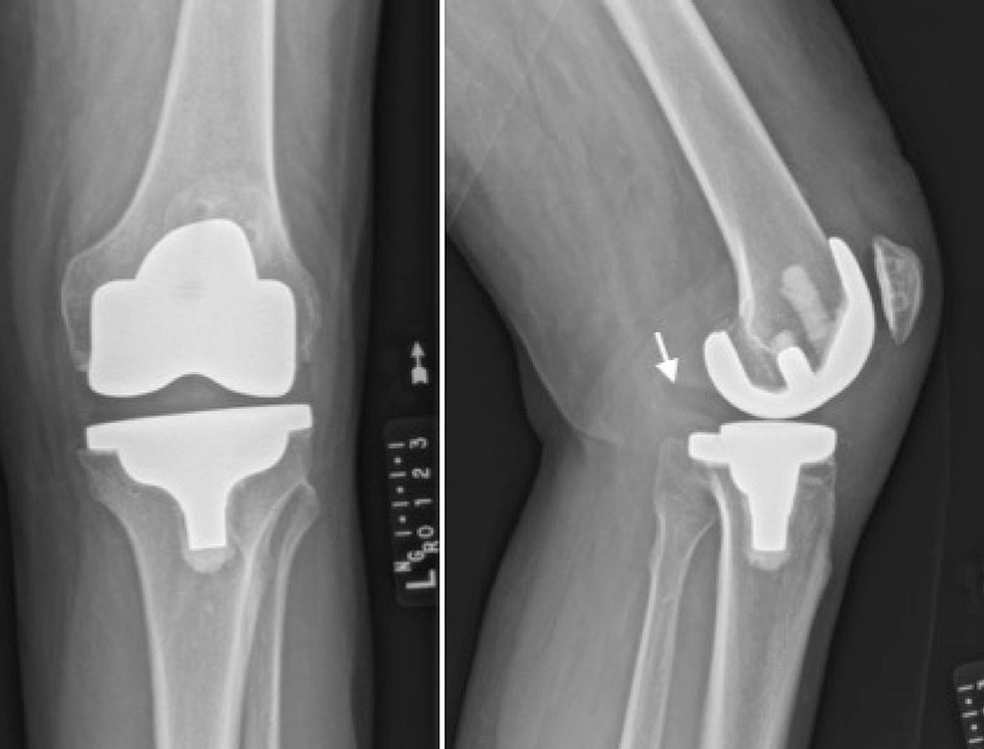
Anteroposterior (left) and lateral (right) radiographs obtained at the 6-week postoperative visit showing posterior dislocation of the tibial polyethylene with asymmetrical joint space.
